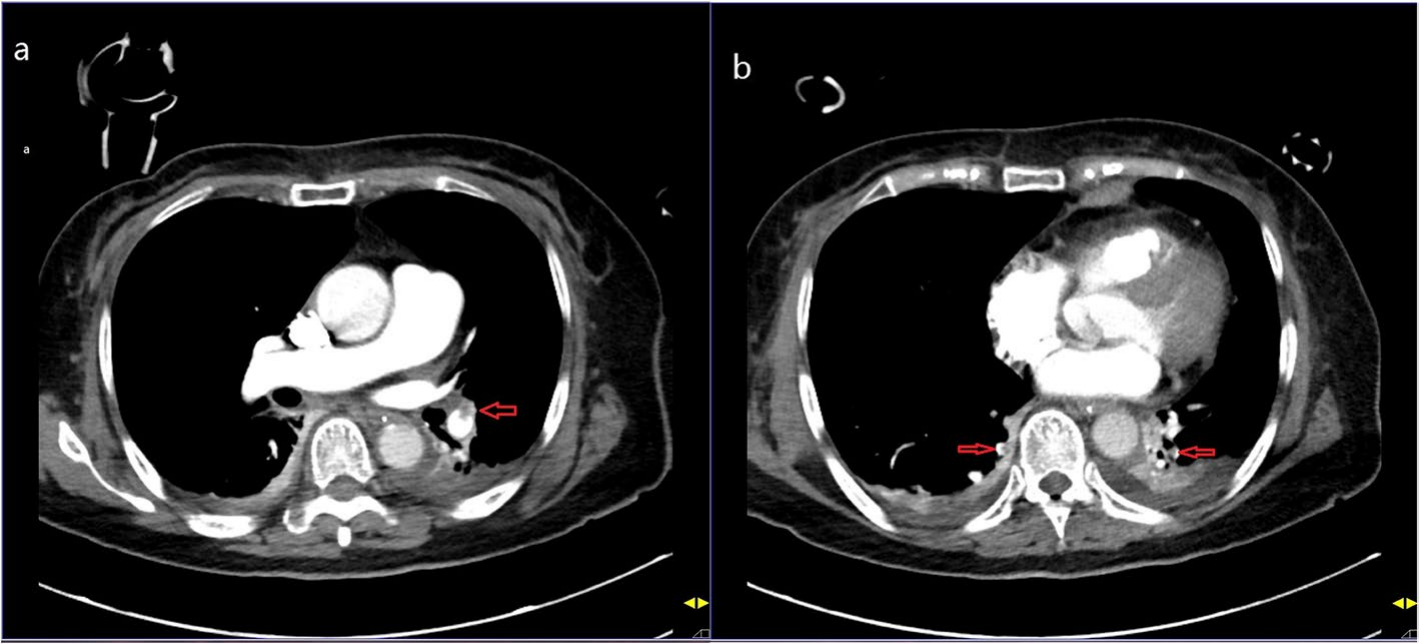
Computed tomography pulmonary angiography shows extensive thrombus burden in segmental branches of pulmonary artery. a: thrombus (red arrow) in left lower pulmonary artery trunk; b: thrombi (red arrows) in posterior basal segment of both lower lobes
